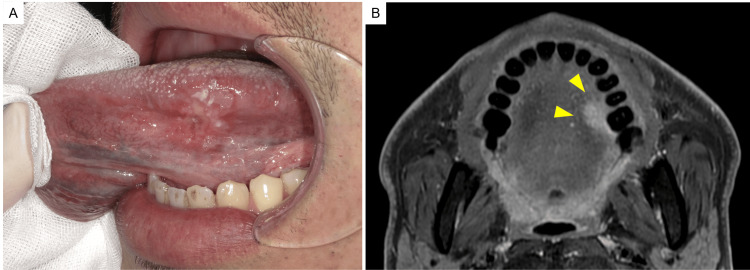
Primary disease at the first examination An unsmooth ulcer on the left side of the tongue on the first examination (A). Contrast-enhanced magnetic resonance imaging (MRI) showing an enhanced tumor in left side of tongue (arrow head) (B).
